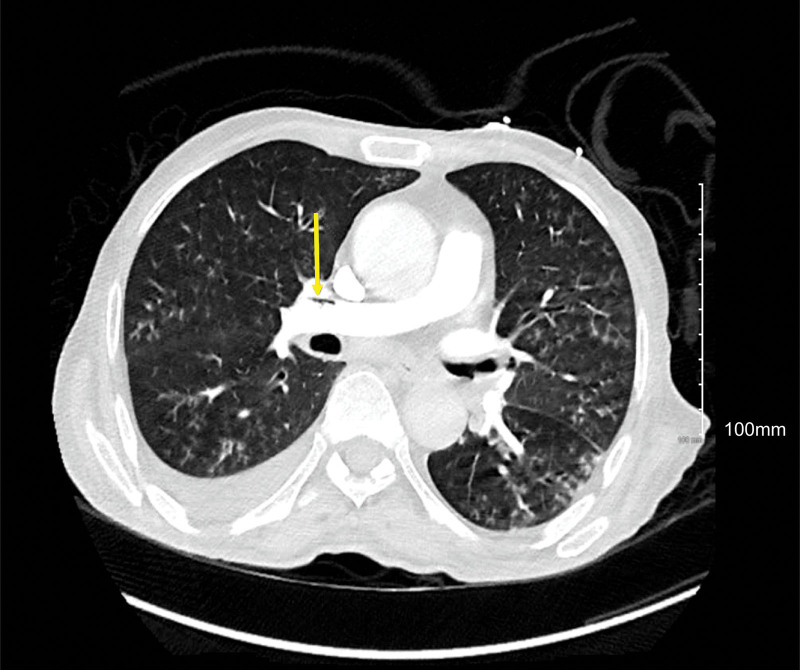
Positive chest CT scan for distal right main pulmonary artery air bubbles. CT = computed tomography.
